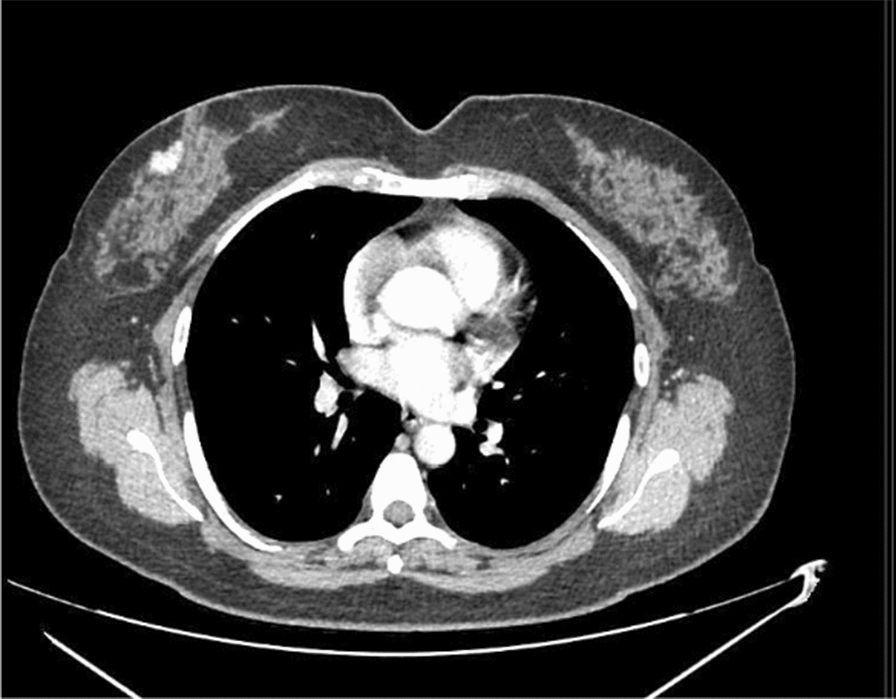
Thoracic CT (axial plane) showing the presence of right breast cancer
